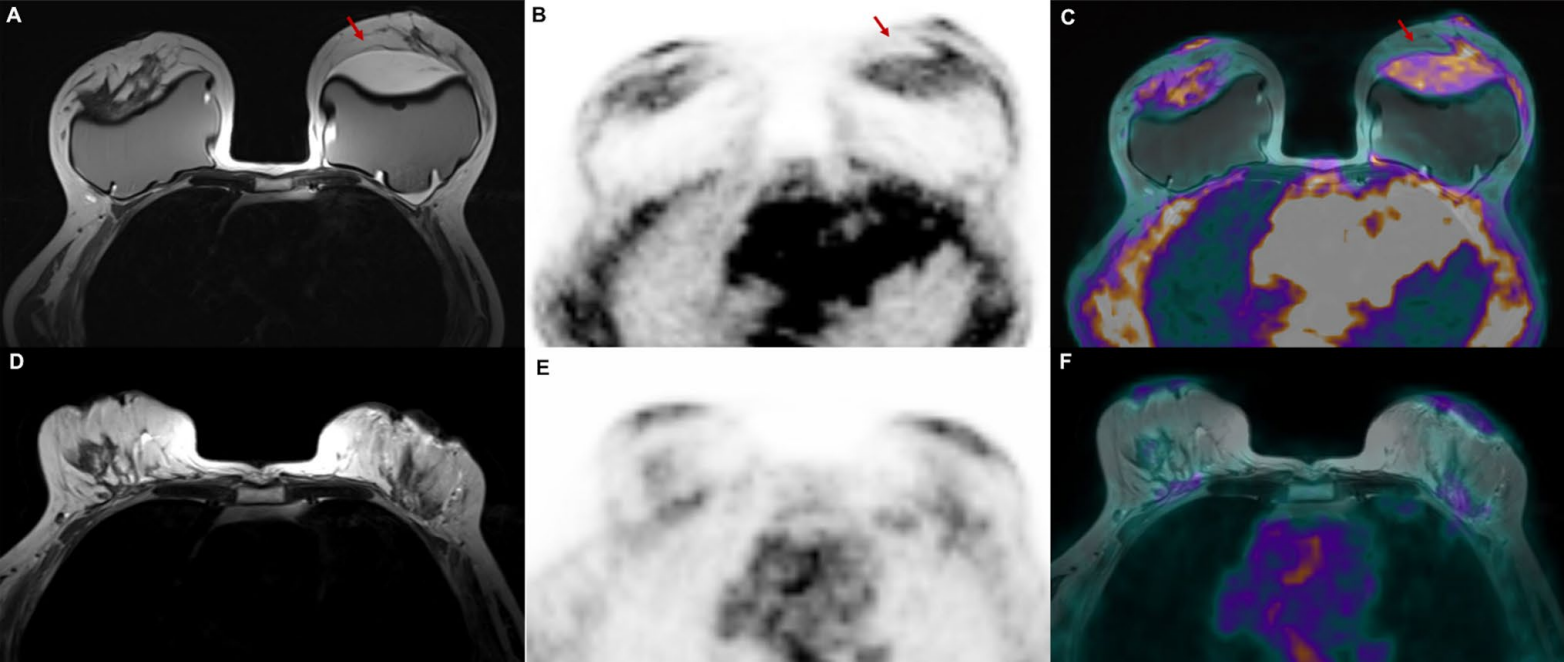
T2-weighted MR axial images (a, d). PET axial images (b, e). PET/MRI axial fused images (c, f). a–c A moderate fluid collection is appreciable around the left breast implant (a, arrow). The fluid collection also showed mild 18F-FDG uptake (b, c arrow). d–f Post-surgical follow-up examination performed after bilateral implant and capsule removal. No significant tracer uptake nor pathological enhancement over breast tissue are detectable
